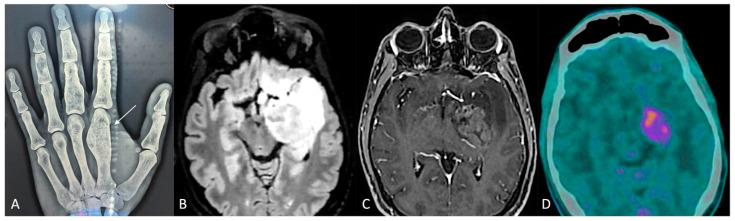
(A) Radiograph of the left hand: enchondroma affecting the first metacarpal bone (white arrow). (B,C) Preoperative brain MRI, axial slices, (B) fluid-attenuated inversion recovery (FLAIR), and (C) contrast-enhanced sequences. (D) PET-CT scan.
